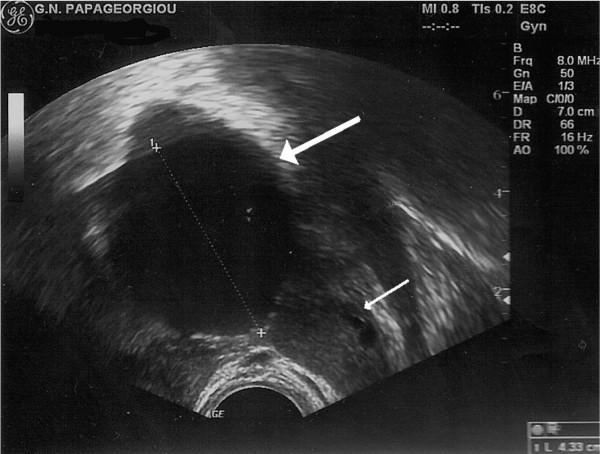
Thick arrow: cystic structure missdiagnosed as para-ovarian cyst in ultrasound. Thin arrow: left ovary.
